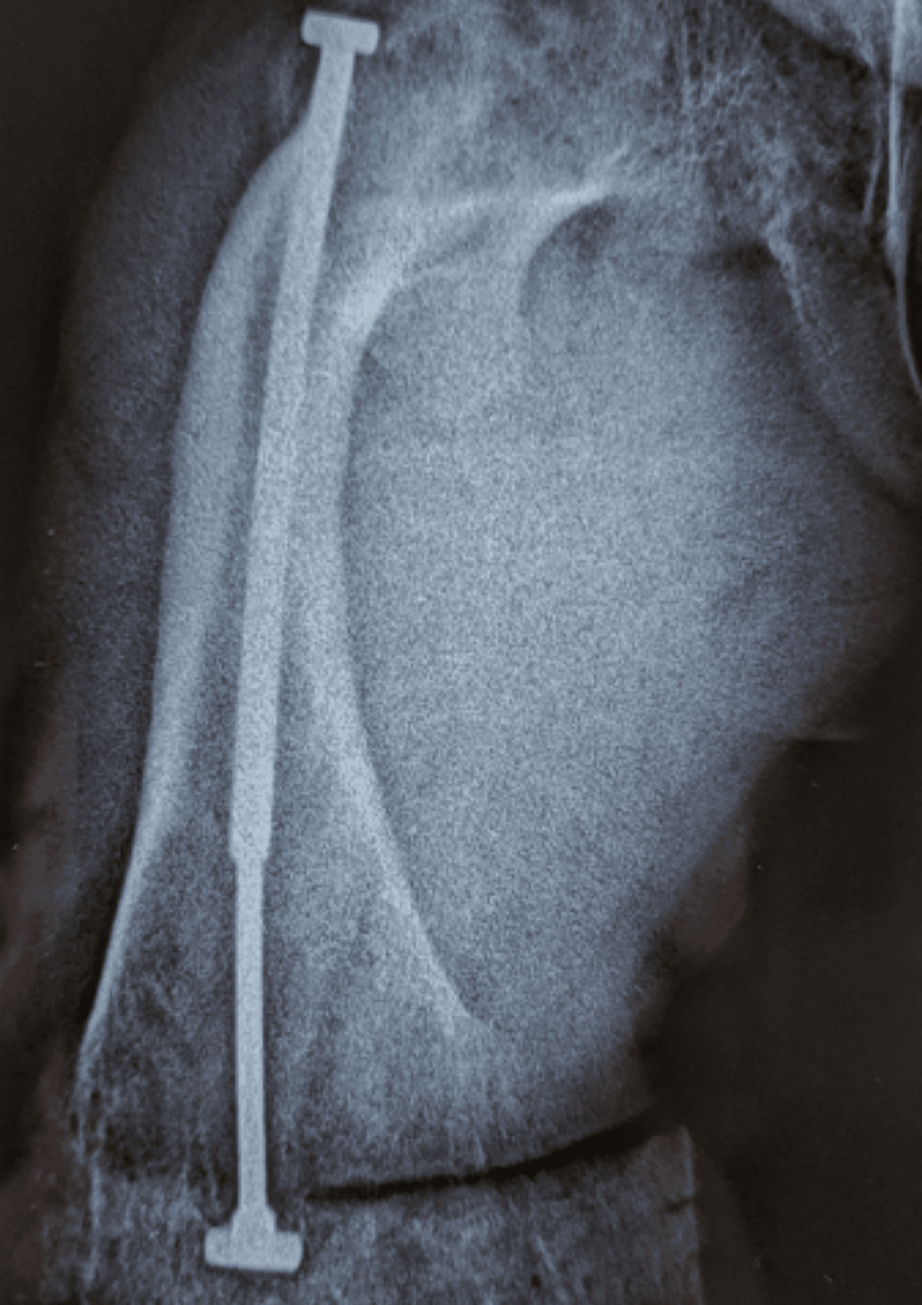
Radiograph demonstrating successful intramedullary rodding with good epiphyseal fixation This fixation allowed the femur to remain protected throughout growth without further deformity or fracture.
